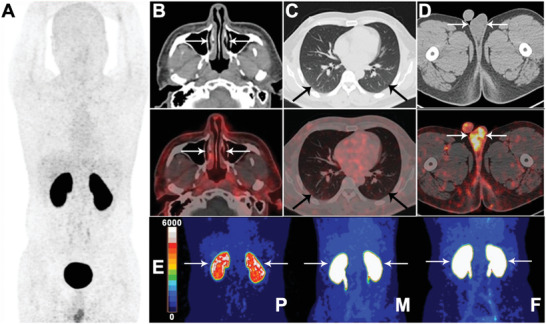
Typical PET imaging of the volunteer recovered from COVID‐19 infection. A 38‐year‐old male infected with COVID‐19 and recovered was included in the study. A) The whole body PET image and B–D) axial PET/CT images of nasal, lung, and testis at 90 min post‐injection were shown. The renal cortex showed obviously lower uptake of ^68^Ga‐HZ20 than healthy male and female volunteers (E; MIP with the same scale bar, P = Patient, M = Male, F = Female). The SUV_max_ of renal cortex were lower than healthy volunteers (SUV_max_ of 11.59 vs 22.18 ± 1.78 for the left kidney, 18.40 vs 25.67 ± 1.39 for the right kidney). The gallbladder and testis showed higher uptake than healthy volunteers (SUV_max_ of 4.32 vs 2.14 ± 0.39 for gallbladder, 8.79 vs 4.51 ± 0.54 for the right testis, 8.65 vs 4.58 ± 0.40 for the left testis), and the SUV_max_ of other organs were listed in Figure 3D compared with the healthy volunteers. The arrows indicate the nasal mucosa in (B), the lungs in (C), the testes in (D), and the kidneys in (E).
